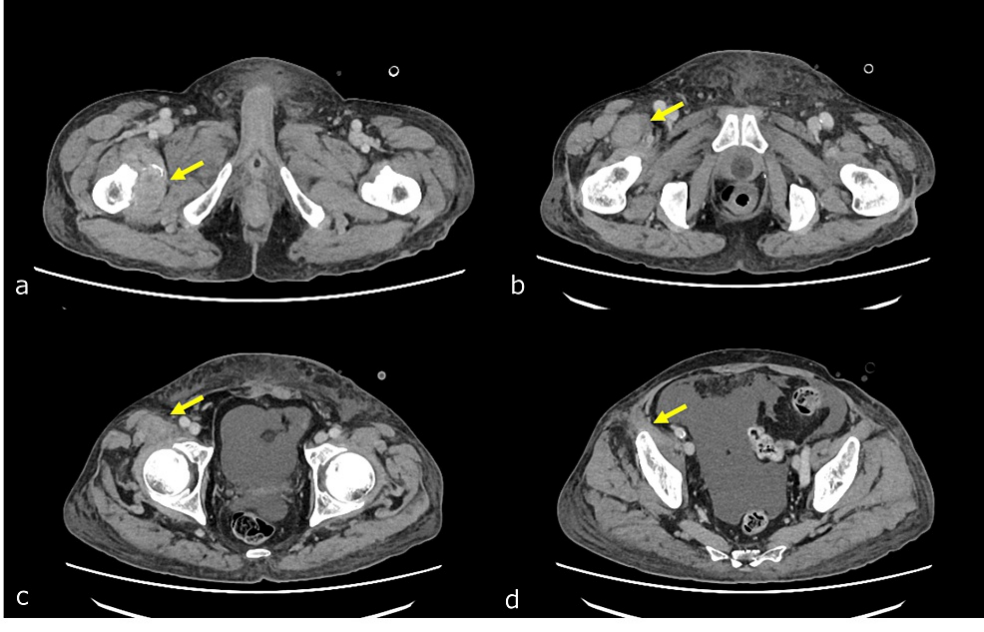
Pelvic CT scan Bone metastasis was observed in the right femoral lesser trochanter (a, indicated by the yellow arrow), along with infiltration and muscle thickening near the right iliopsoas muscle transversion (b-c, indicated by the yellow arrow), and muscle thickening extending to the right psoas muscle near the iliac crest (d, indicated by the yellow arrow).
